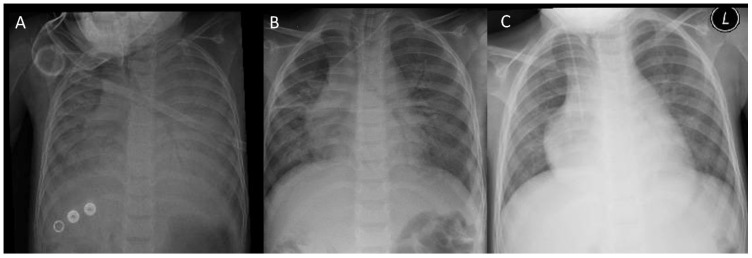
Patient 1: (A) frontal CXR showing bilateral opacities silhouetting bilateral hemidiaphragm and cardiac borders; (B) follow-up CXR after 2 days showing significant improvement with minimal residual patchy infiltration involving both lungs; (C) CXR on day 5 of admission showing complete resolution of the lung opacities. L = left, CXR = chest X-ray.
